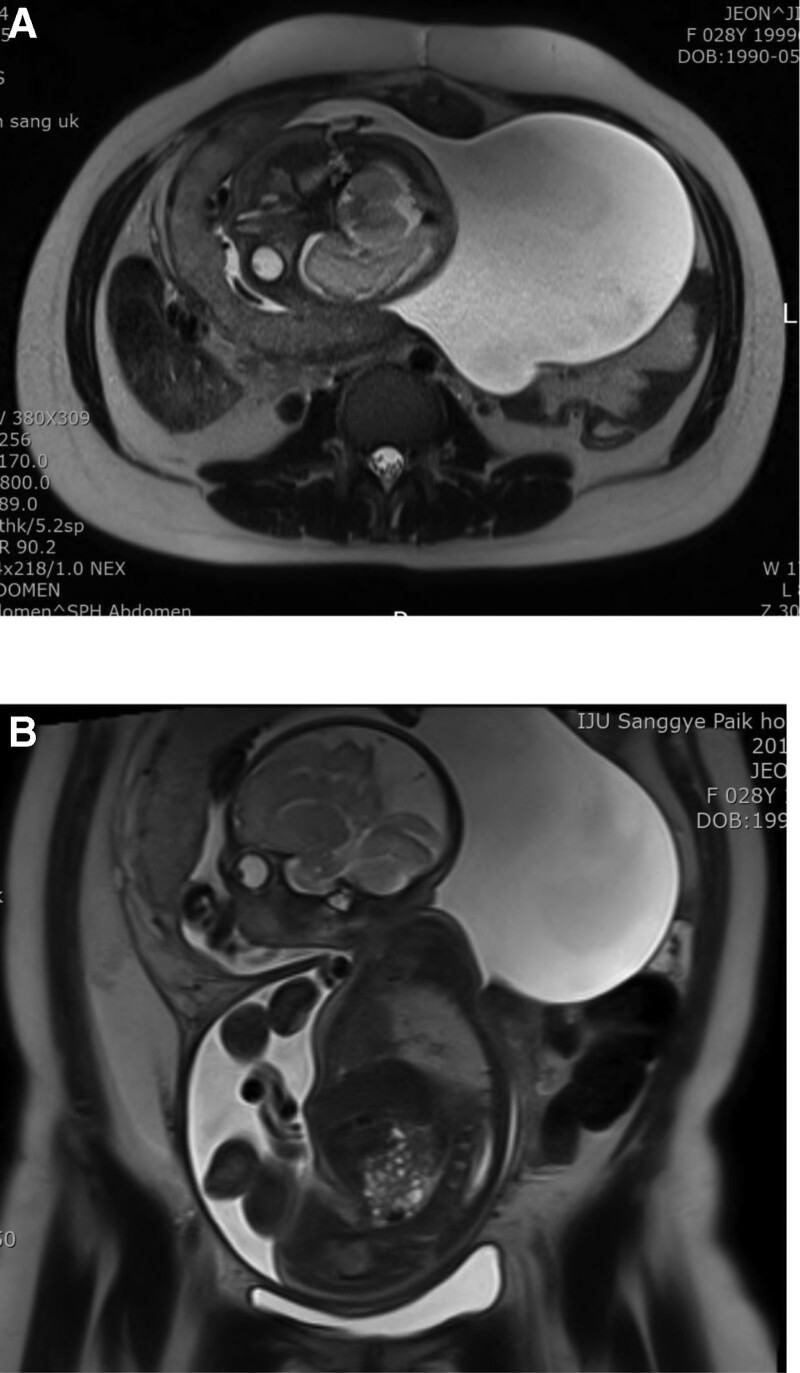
Magnetic resonance imaging of patient before and after uterine rupture. (a) Axial view showing no evidence of appendicitis. (b) Several hours later, coronal view shows the fetal head and the amniotic sac protruding through a large uterine defect.
